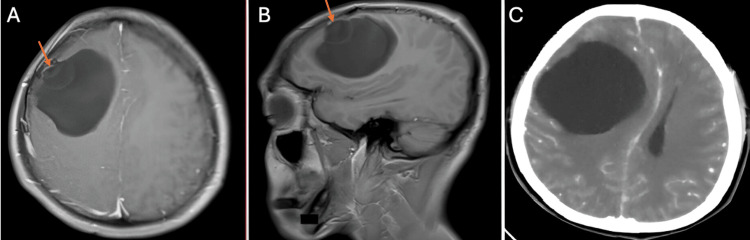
MRI showing a giant cysticercus cyst with scolex inside (orange arrow) (A,B). Head CT showing the cystic mass exerting a severe mass effect, generating deviation from the midline (C) MRI: magnetic resonance imaging; CT: computed tomography
